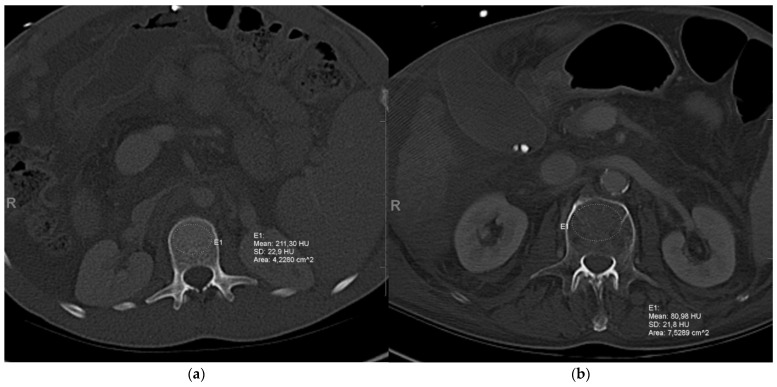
Assessment of bone mineral density (BMD) on routine CT scans. BMD was measured on routine CT scans upon ICU admission in the anterior portion of the trabecular space in the upper one-third of the L1. Two exemplary CT scans of patients with (a) high (221.3 HU) or (b) low BMD (80.98 HU) are shown.
